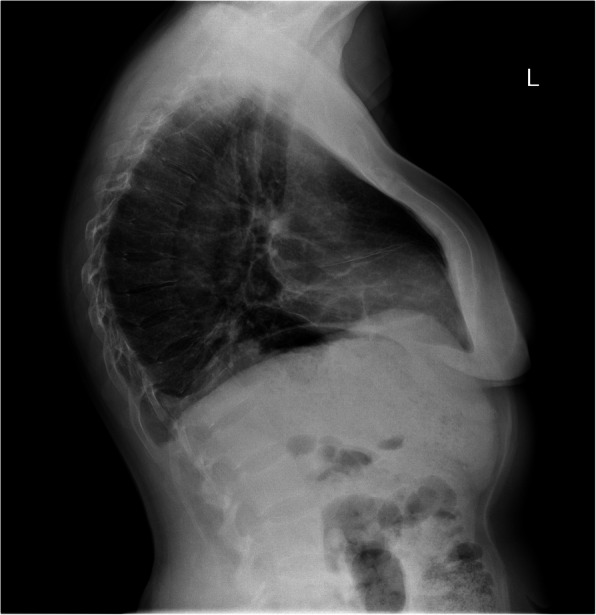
Chest radiograph showing marked kyphosis
